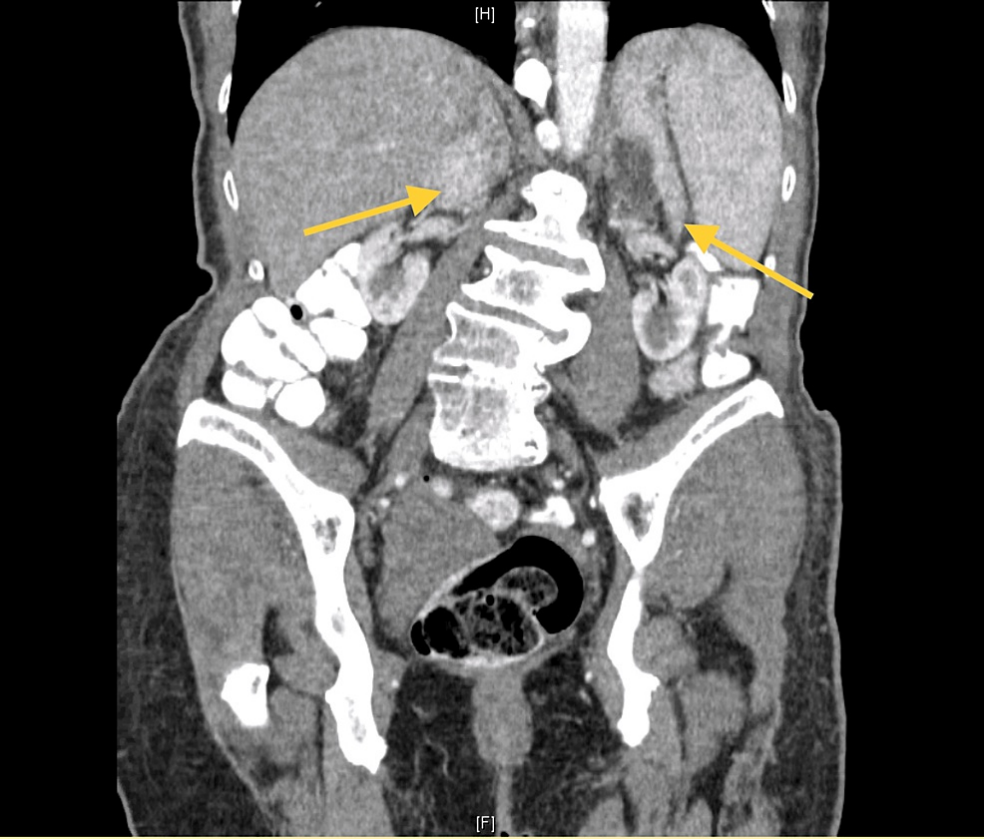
CT of the abdomen with contrast showing bilateral adrenal myelolipomas (coronal view) CT: computed tomography
